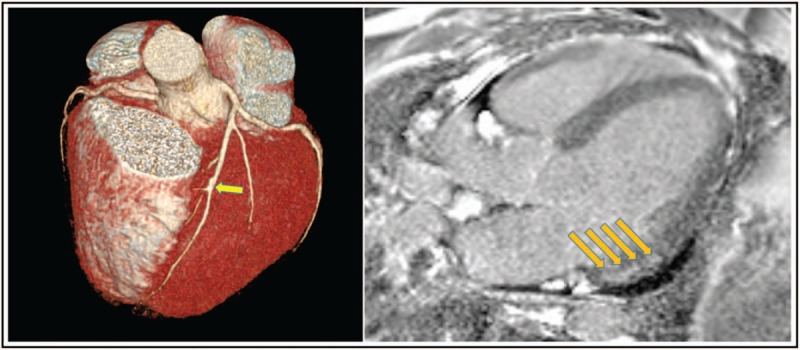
Cardiac computed tomography (left) and magnetic resonance (right) images of a coronavirus disease 2019 patient with chest pain and elevated cardiac troponins (1088-54 ng/l; normal range <15 ng/l) and left ventricular ejection fraction 44%. Cardiac CT shows open coronary artery, only a minor calcified lesion in mid-LAD (arrow). Cardiac MR shows late gadolinium enhancement (arrows) in the left ventricular inferolateral wall, mid-myocarrdium/subepicardial, suggesting subacute perimyocarditis. CT, computed tomography; LAD, left anterior descending artery; MR, magnetic resonance.
